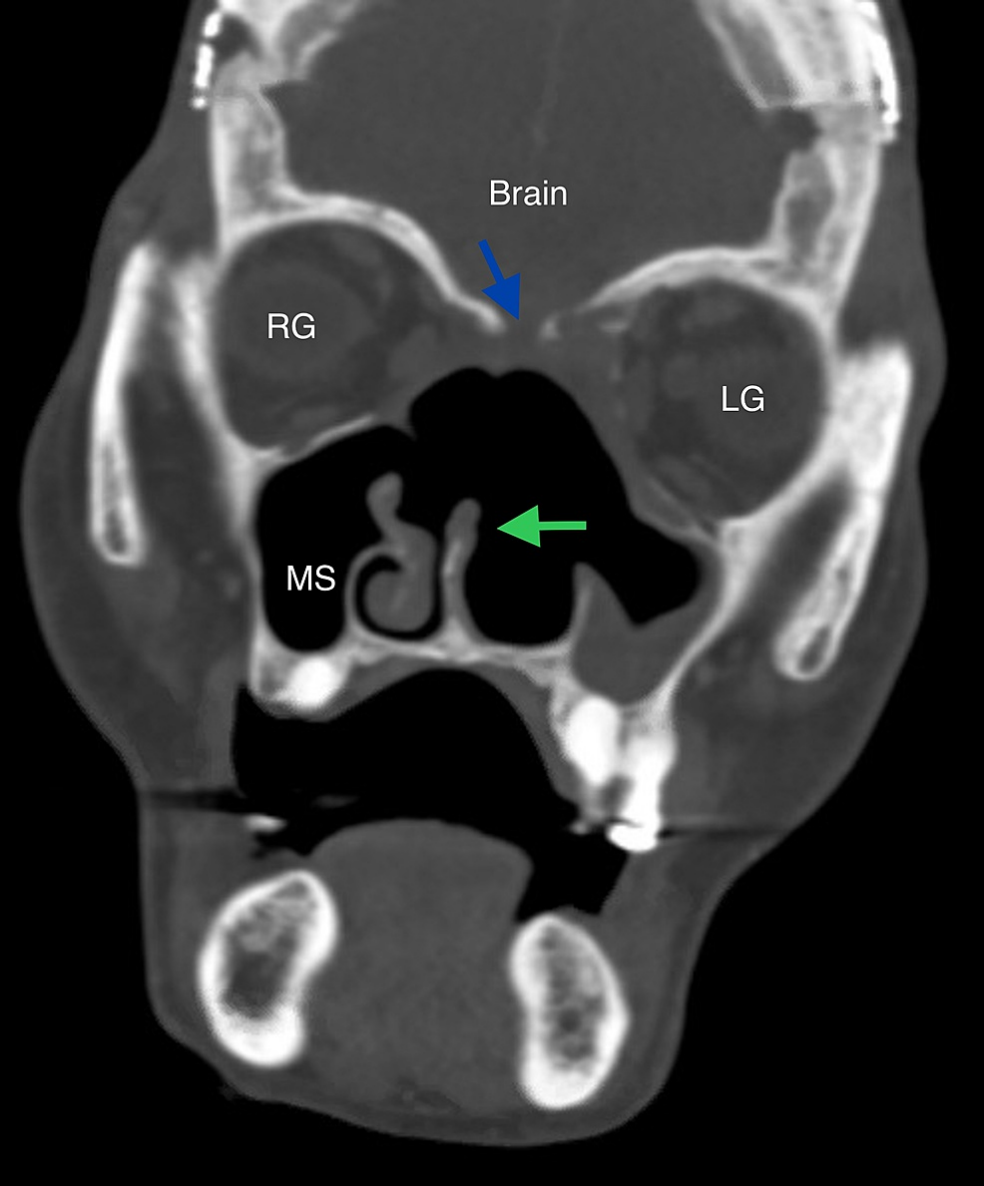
CT scan, bone window, coronal view, at the level of the orbits, one year after resection. Blue arrow once again indicates bony skull base defect lateral to the cribriform plate. Right globe (RG) and left globe (LG) no longer demonstrate proptosis. Green arrow indicates remnant of nasal septum. Right maxillary sinus (MS) and abutting right inferior turbinate are the only remaining landmarks in the nasal cavity.
